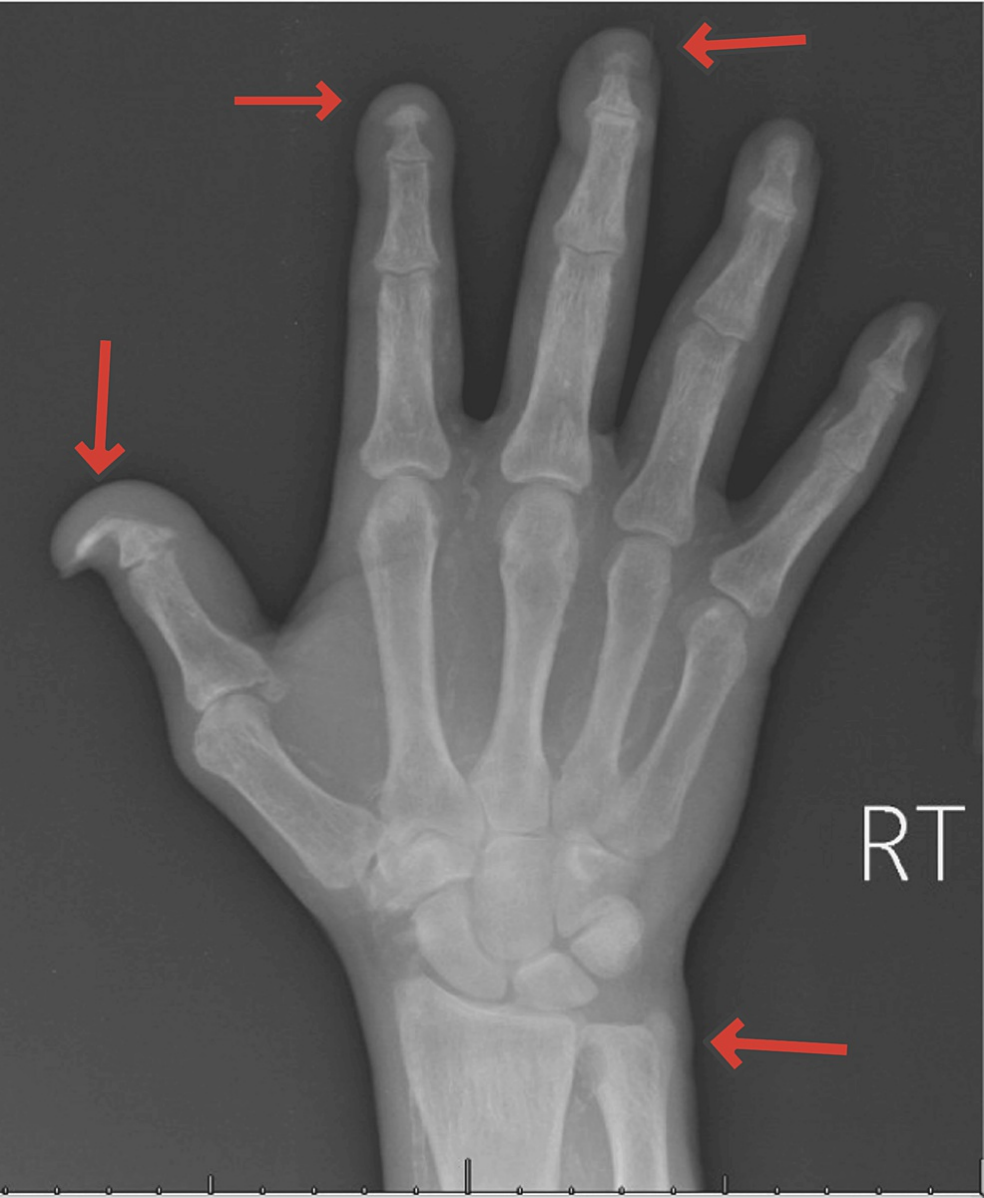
Three-view right hand X-ray: resumption versus osteolytic process at the distal ulna at the distal radioulnar joint, as well as chronic resumption and/or post-traumatic changes of the first, second, and third distal phalanges (arrows)
